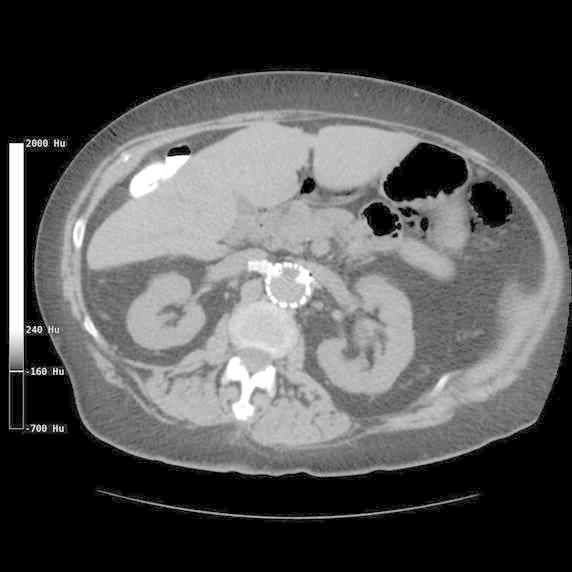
Repeat CT at 4 months post discharge showing complete resolution of the hepatic abscess.
